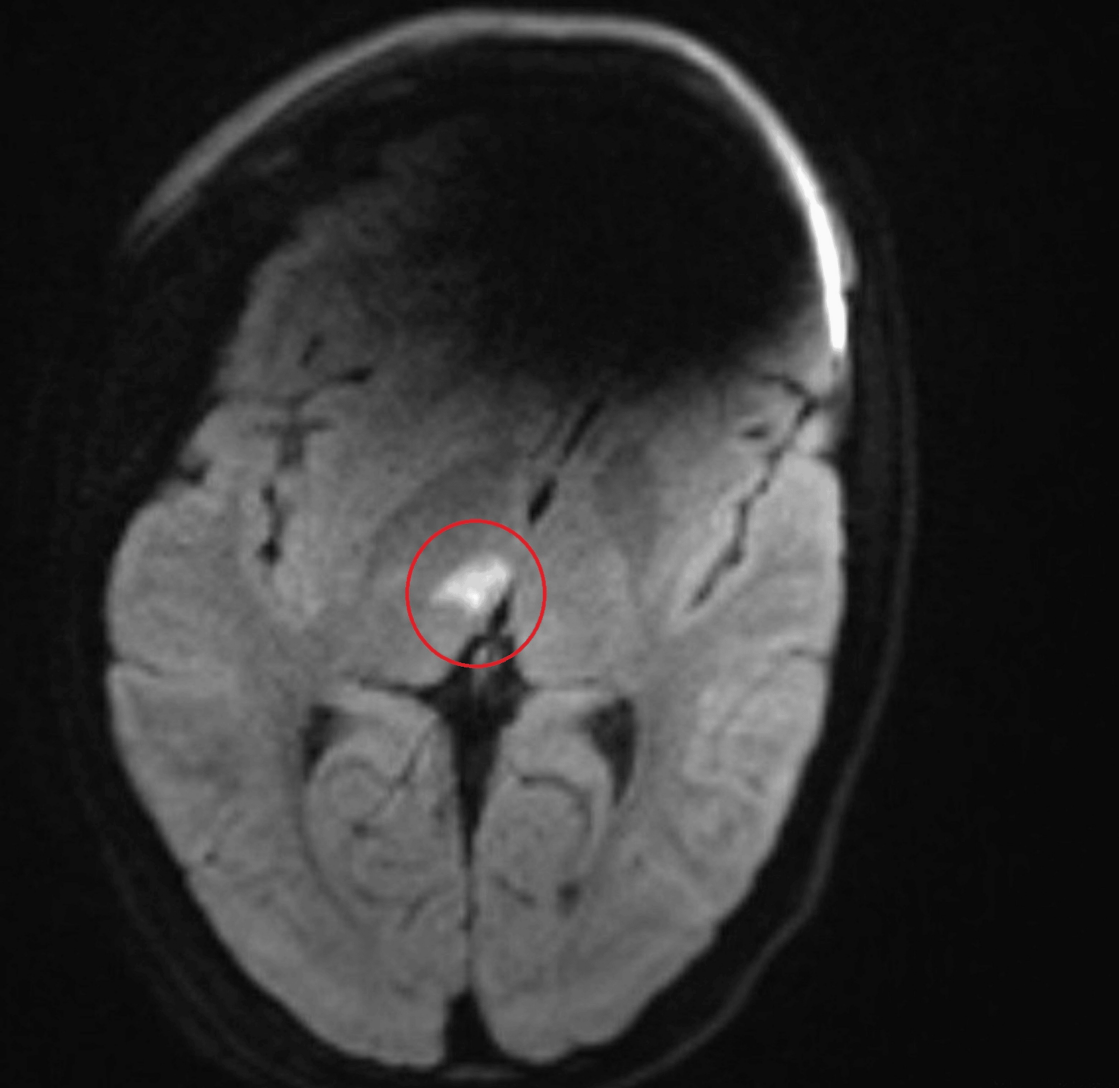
MRI brain, DWI sequence, showing an area of restricted diffusion in the right thalamus, consistent with an acute ischemic infarct (outlined in red).
